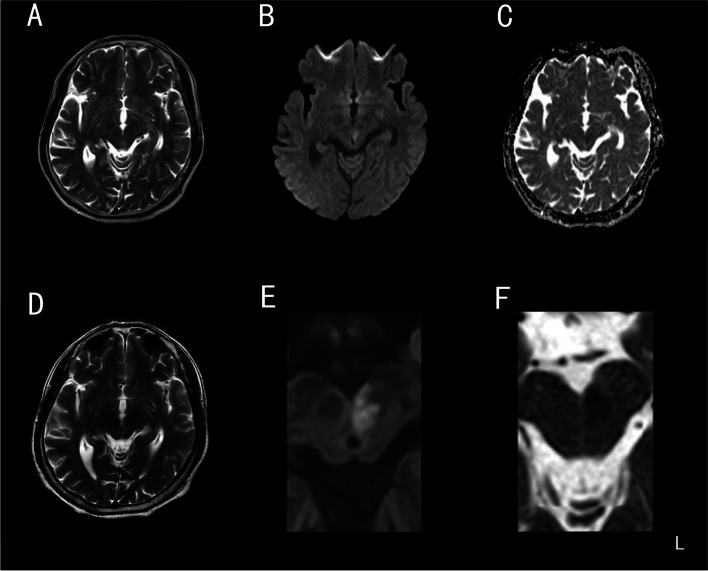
The mesencephalic periaqueductal slice T2WI (A), a suspicious restriction in conventional DWI (B), correlated apparent diffusion coefficient map (C), a corresponding T2WI of the ZOOM DWI (D), a verified restriction in the ZOOM DWI (E) and correlated apparent diffusion coefficient map (F). Abbreviations: L, left
